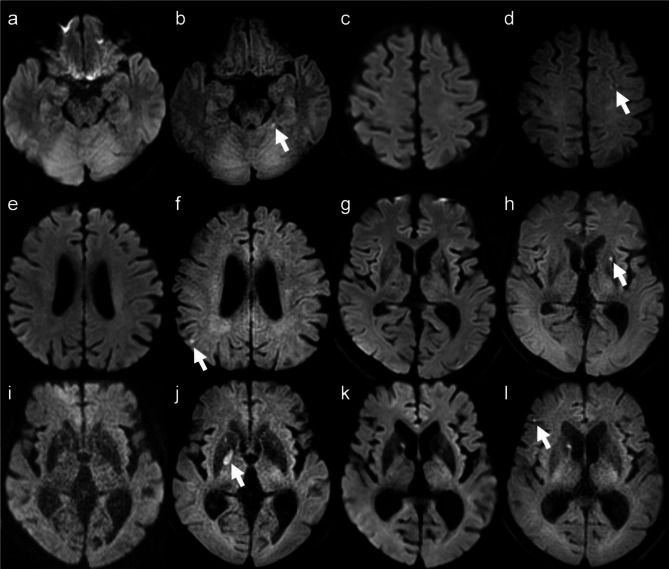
Representative images in patients with acute or subacute infarction. The white arrows indicate infarctions in the cerebellar hemisphere near the cerebellar tentorium (a, b), left frontal cortex (c, d), right parietal lobe (e, f), left putamen (g, h), right globus pallidus (i, j), and right frontal cortex (k, l). SS-EPI DWI (a, c, e, g, i and k); TGSE-BLADE DWI (b, d, f, h, j and l). The lesions are detectable only on TGSE-BLADE DWI but are unclear on SS-EPI DWI due to proximity to cortex, cerebellar tentorium, or hemosiderin deposition in the basal ganglia.
